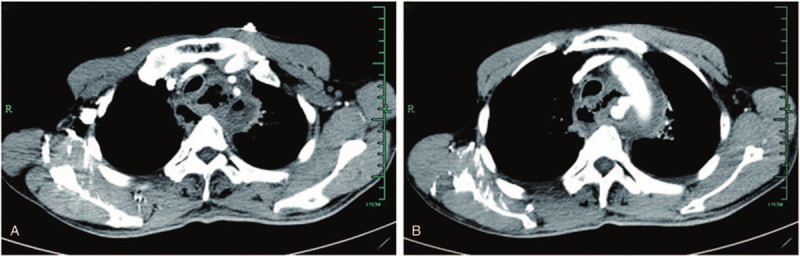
Representative images of case 1. (a) a swollen thoracic esophagus with several mediastinitis, pneumomediastinum on contrast-enhanced chest CT. (b) a pseudoaneurysm located in the aortic arch on ontrast-enhanced chest CT.
